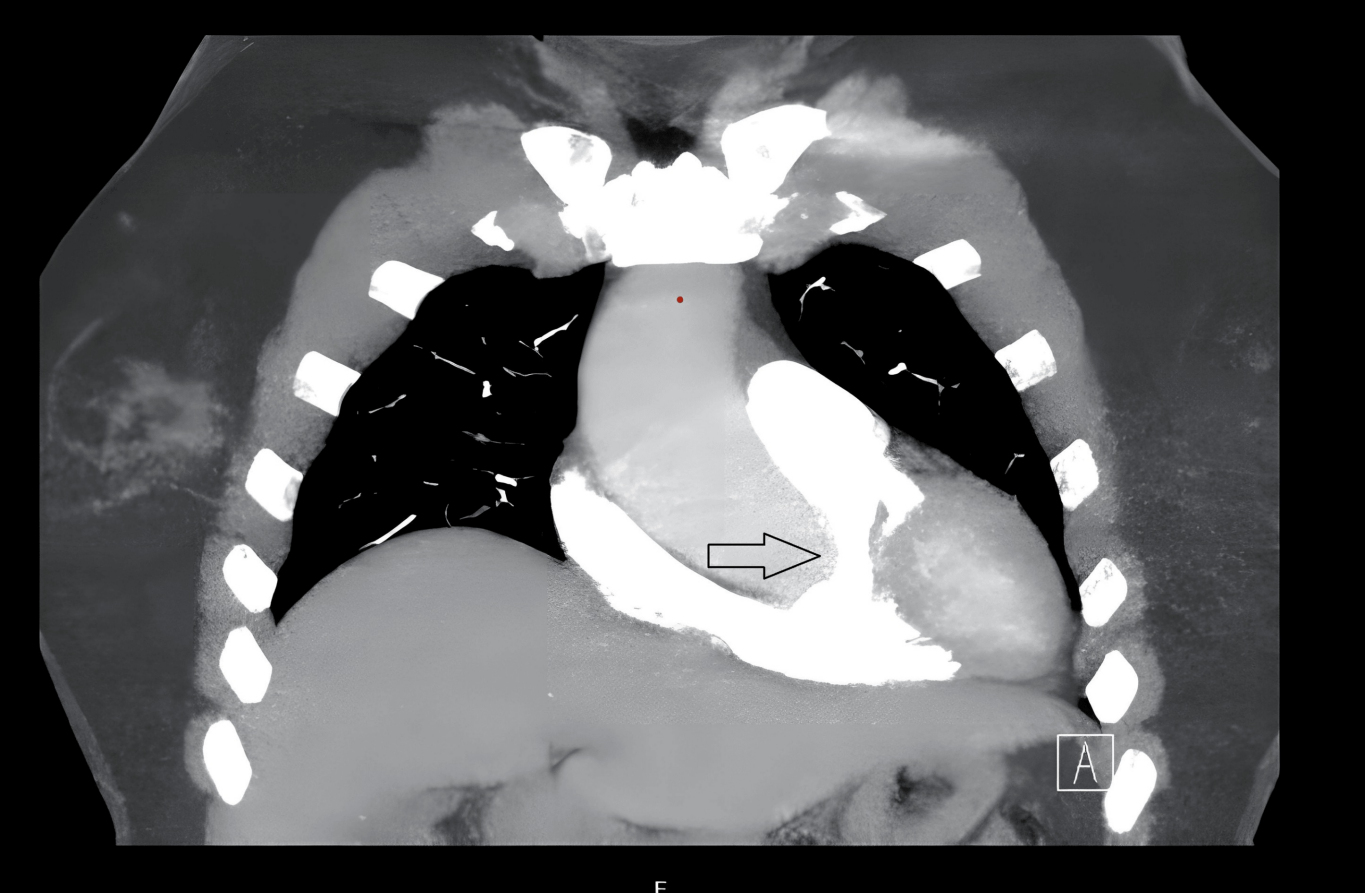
CT angiogram aorta and the arrow depicts aneurysmal dilatation of the ascending aorta that measures 4 cm.
